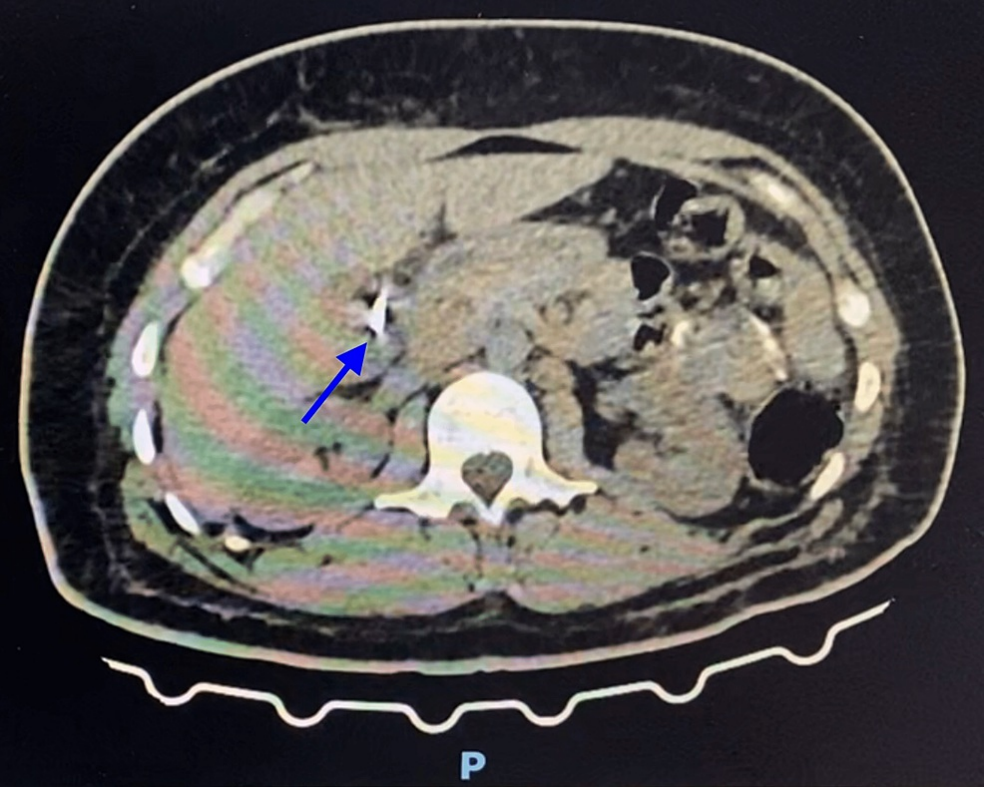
CT scan of the abdomen The image shows a small amount of liquid collected on the hepatic bed, extending medially adjacent to the VI hepatic segment (marked by the blue arrow).
